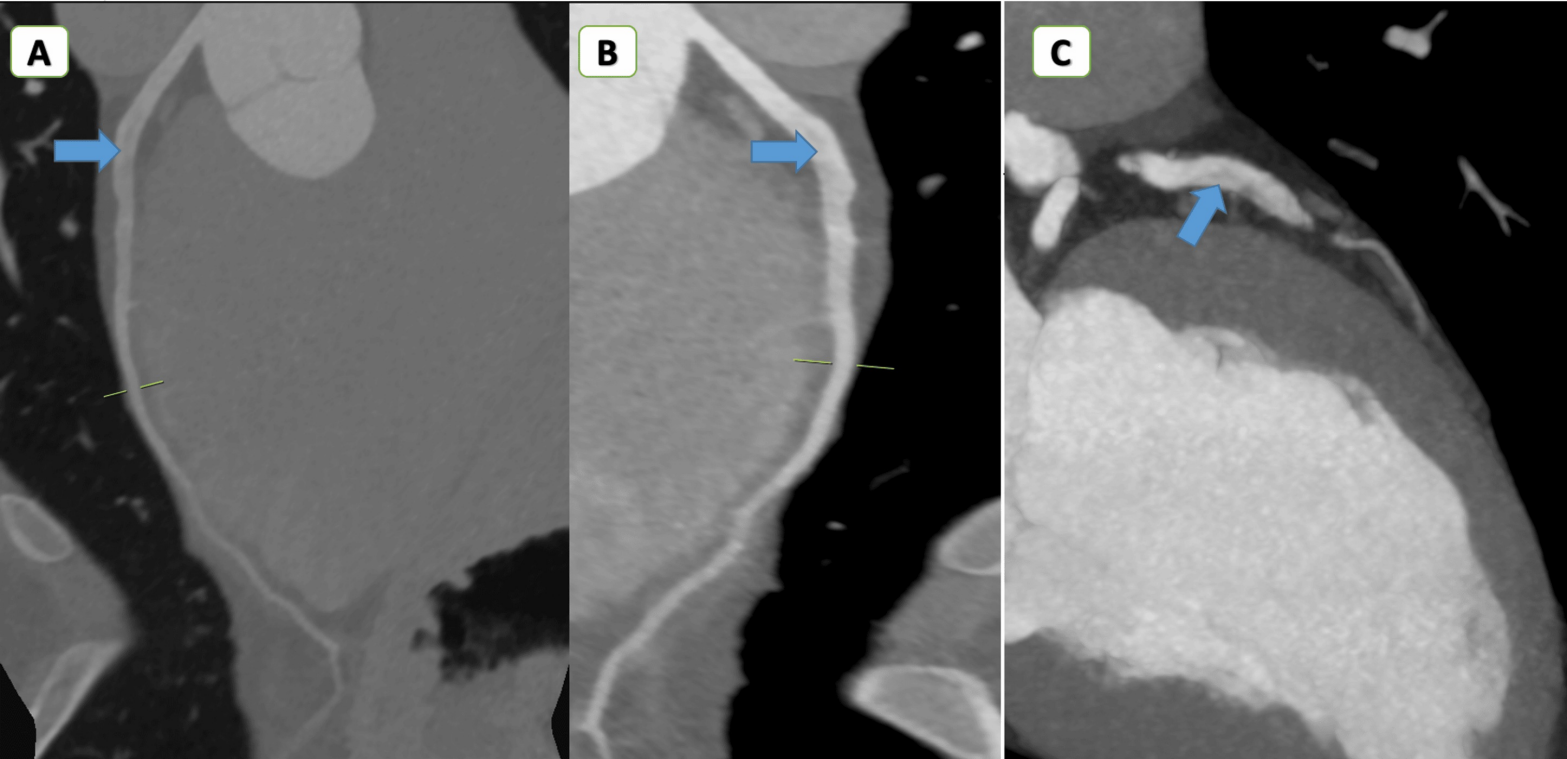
CTCA showing LAD with a dissection flap. CTCA, computed tomography coronary angiography; LAD, left anterior descending artery
